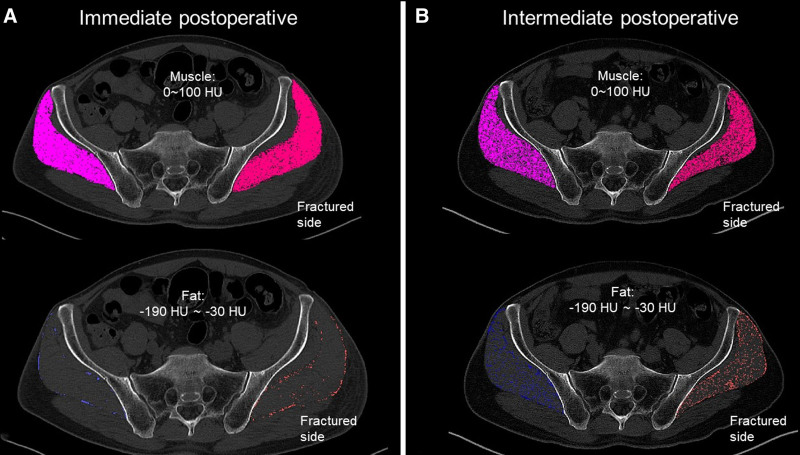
Axial computed tomography (CT) slice of the muscle (top) and adipose tissue (bottom) at the fracture (crimson/red) and uninjured sides (violet/blue) during the immediate (A) and intermediate postoperative periods (B). Over time, a reduction in the area of muscle tissue and a corresponding increase in the area of adipose tissue can be observed.
